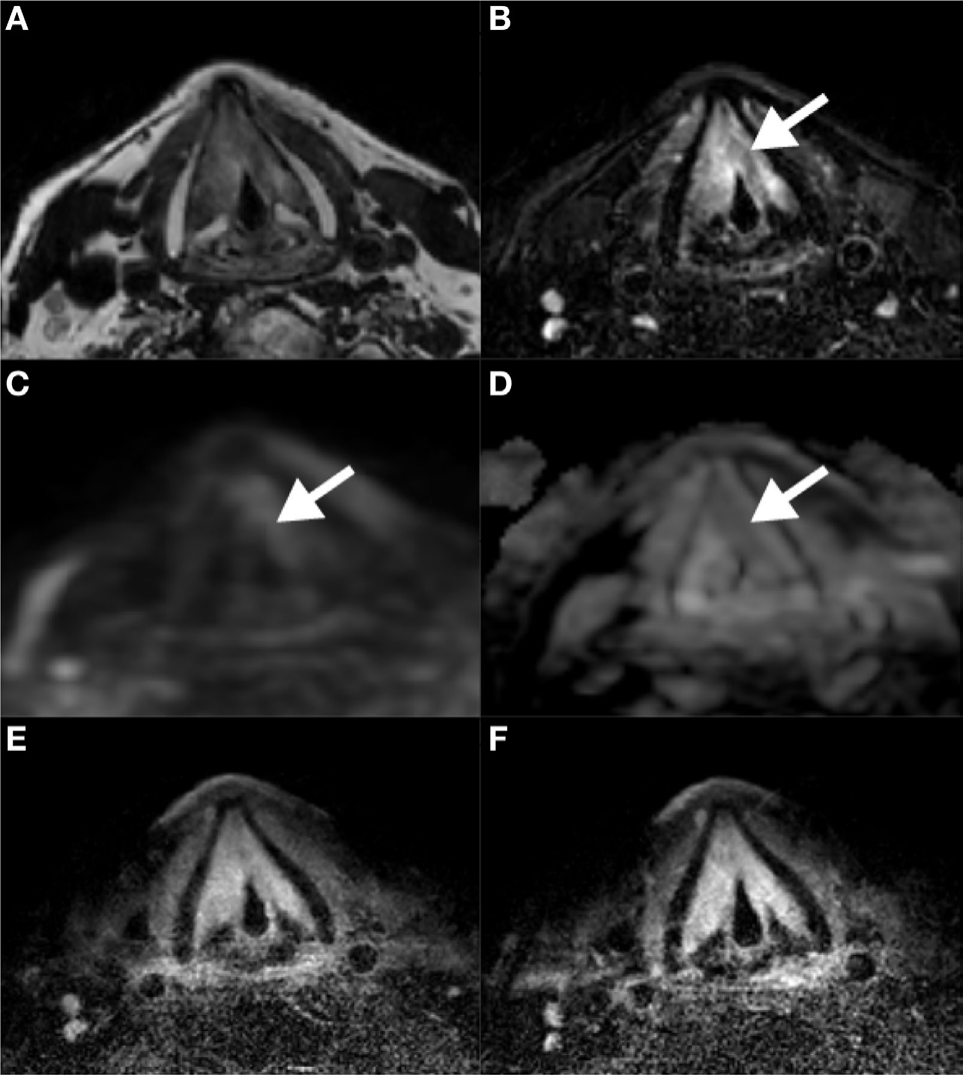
Magnetic resonance imaging scans 8 months after radiation therapy for a T1b glottis carcinoma. Increased signal intensity on T2-weighted scans without (A) and with (B) fat suppression in both vocal cords, together with the absence of diffusion restriction on DWI/ADC (C,D) is compatible with posttreatment edema. A biopsy in the superficial area corresponding to intermediate signal on T2 and diffusion restriction on the left vocal cord revealed tumor recurrence. Contrast-enhanced T1-weighted scans at two different time points show gradual enhancement (E,F).
